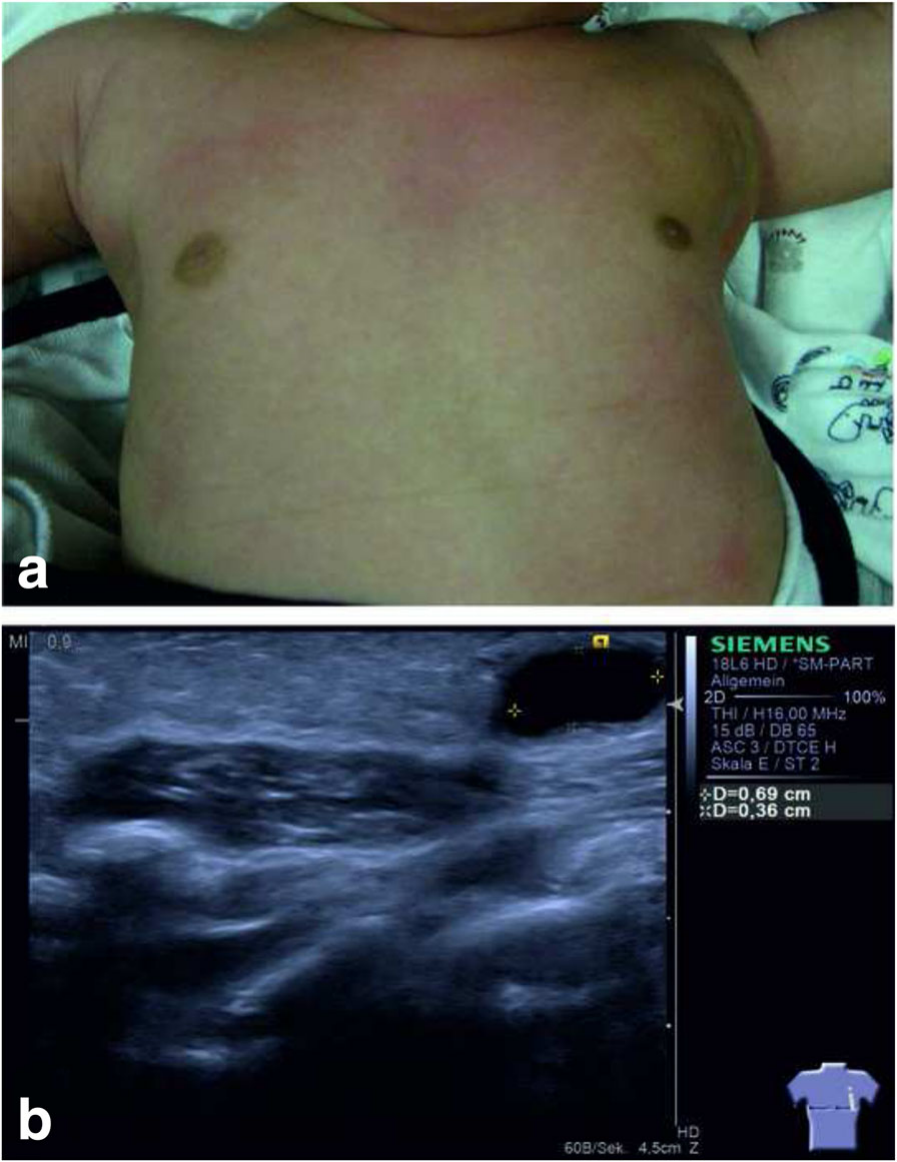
a Nearly total remission of the tumor three weeks after sclerosant therapy. b B-Mode ultrasound showing a singular small anechoic cyst as a residuum of the CCL 8 weeks after sclerosant therapy
